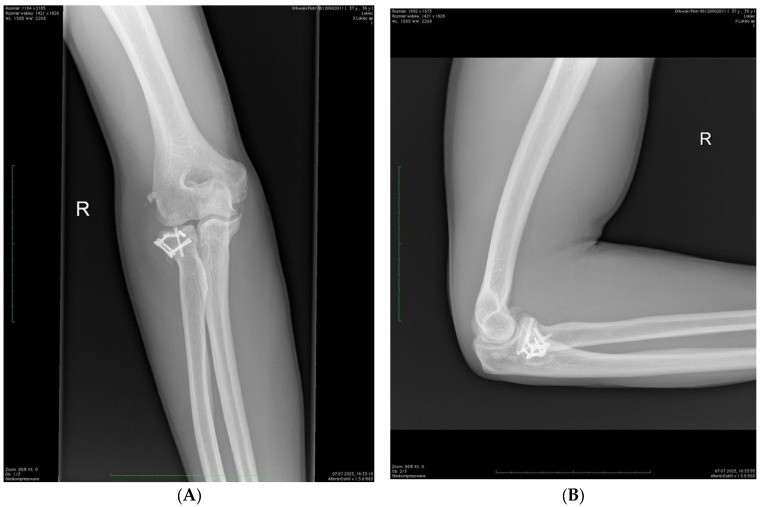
Control X-ray in the same patient 23 months after surgery—AP (A) and lateral (B) projections. Complete bone union and asymptomatic periarticular ossification (Hastings–Graham 1).
